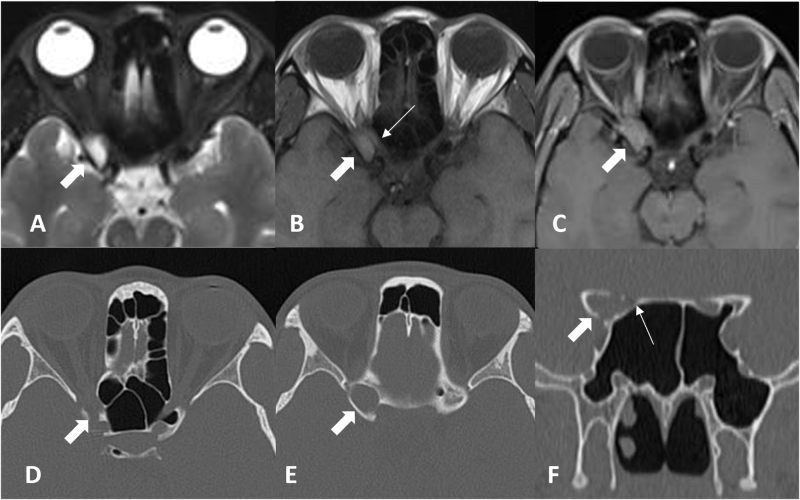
Preoperative MRI and CT results: (A) Axial T2, (B) Axial T1, and (C) Axial T1 with contrast: showing an expansile lesion at the orbital apex (thick arrows) with slightly hyperintense T1 signal, hyperintense T2 signal, and peripheral marginal enhancement on contrast-enhanced T1; thin arrow in (B) indicates the compressed right optic nerve. (D) Axial CT, (E) Axial CT, (F) Coronal CT: showing the expansile mucocele as soft tissue density (thick arrows) with bony thinning of the OS and ACP; thin arrow in (F) indicates the compressed right optic canal.
